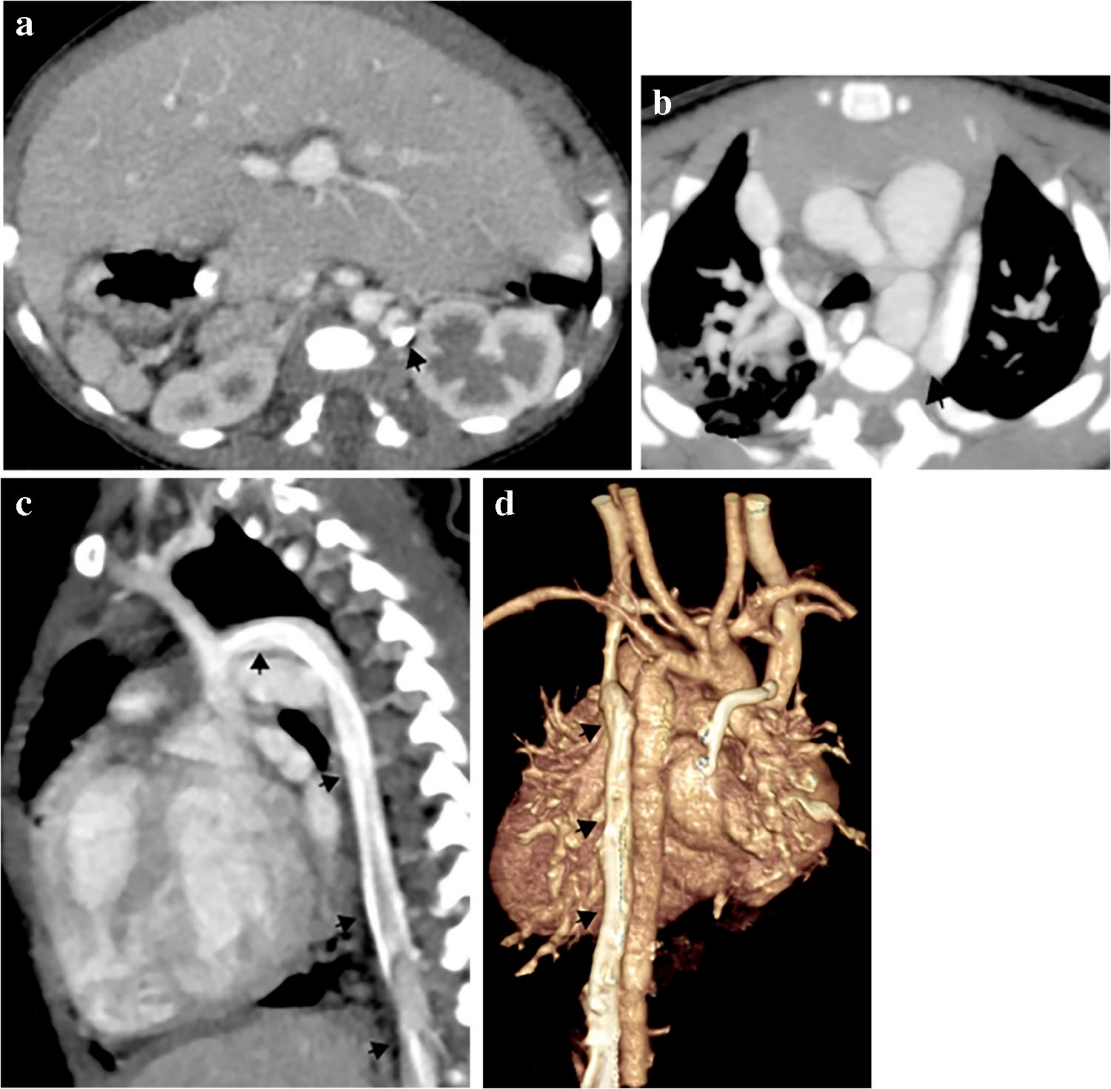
Inferior vena cava (IVC) interruption with hemiazygos continuation in a 5-month-old girl with left isomerism. a–d Axial (a, b), sagittal oblique (c) and volume-rendered (d) computed tomography (CT) images show IVC interruption with hemiazygos continuation draining into the persistent left superior vena cava (SVC) (*arrows*). Note the azygos arch draining into the right SVC, polysplenia on the right side and central liver position. The aortic arch is hypoplastic and there is aortic coarctation
